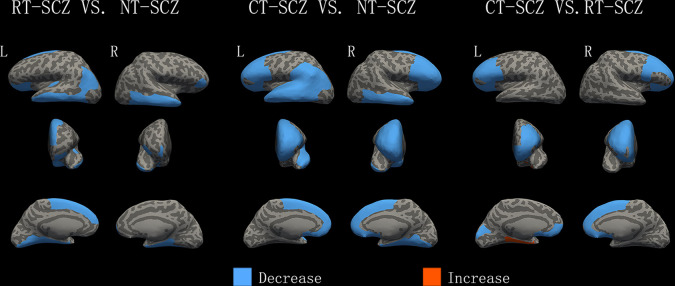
Differences in GM between treated and never treated patients with chronic schizophrenia. Greater cortical volume, surface area, or thickness in treated patients than in comparison subjects are indicated by red/warm color, and lower volume, surface area, or thickness are indicated by blue/cold color. L, left hemisphere; R, right hemisphere; NT-SCZ, never treated schizophrenia patients; RT-SCZ, risperidone-treated schizophrenia patients; CT-SCZ, clozapine-treated schizophrenia patients.
